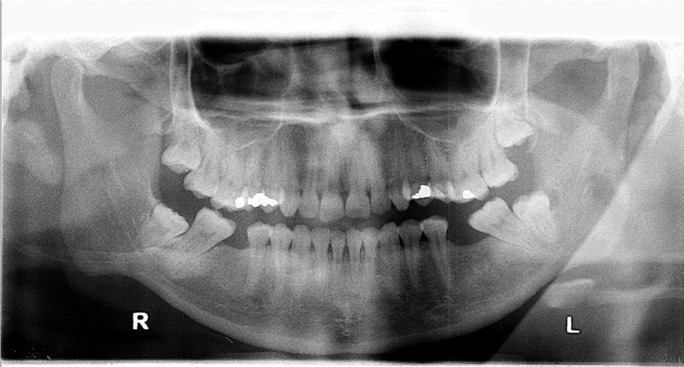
Panoramic X-ray view at initial presentation. Note the irregular pericoronal radiolucency associated to partial bony impacted tooth 3.8 in vertical position (2B Pell and Gregory). Pericoronal distal widened space compatible with paradental inflammatory cyst.
